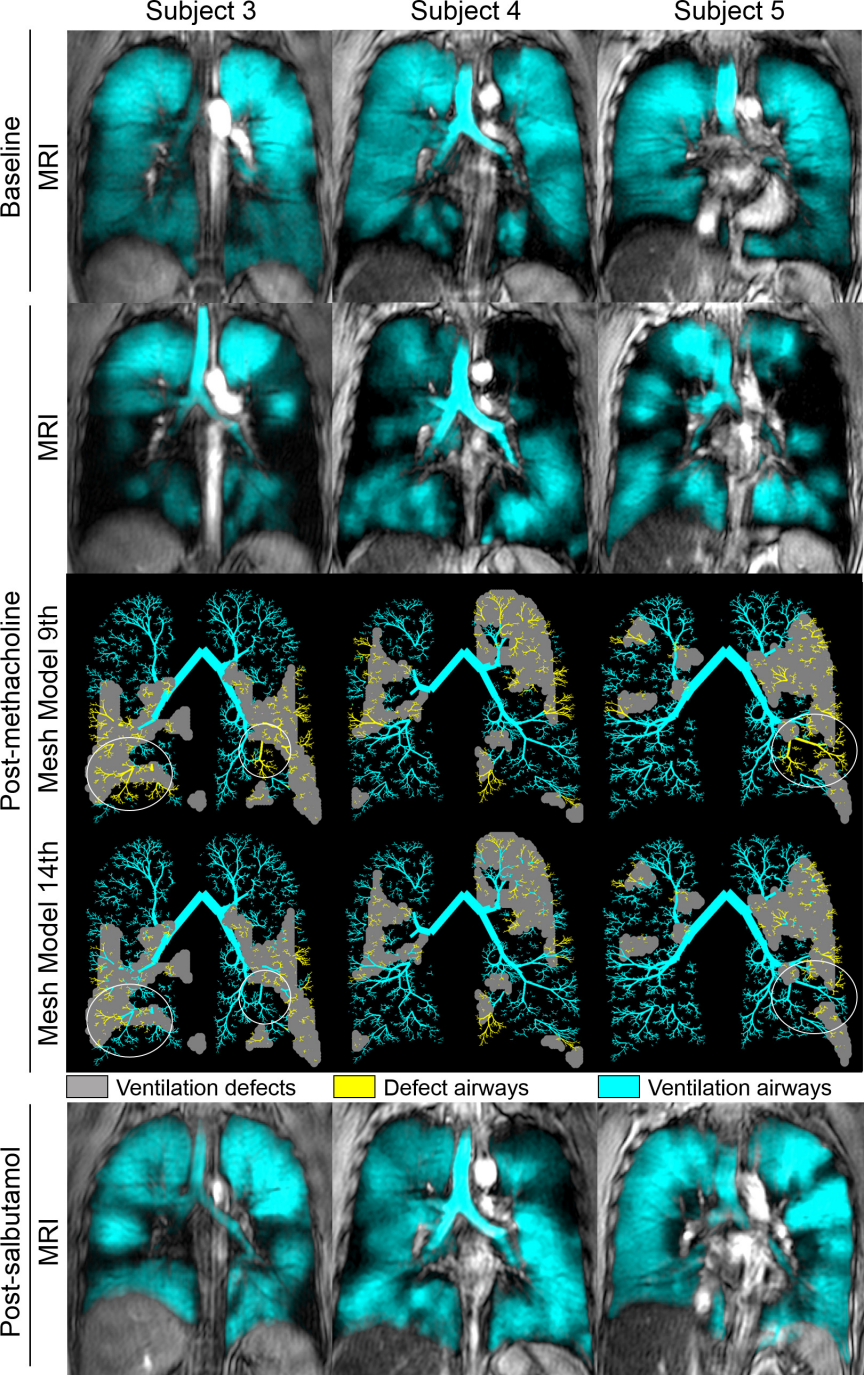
MRI and model‐to‐MRI coregistration for three asthmatics showing colocalization of airway tree and ventilation defects post‐methacholine. Center coronal slice MRI ventilation in blue coregistered to anatomical MRI in gray scale at baseline, post‐methacholine and post‐salbutamol. For each subject, the corresponding post‐methacholine mesh models with closure of airways from 9th (Row 3 across) or 14th generation (Row 4 across) are shown. For the mesh model, gray reflects an MRI ventilation defect, yellow segments are specific to mesh model airways located within a ventilation defect and cyan segments airways were mesh model airways that did NOT lead to ventilation defects. White circles show differences reflected by closure of either the 9th (row 3) or 14th (row 4) generation airways in the model. Subject 3: 36‐year‐old female; baseline/post‐methacholine/post‐salbutamol FEV
